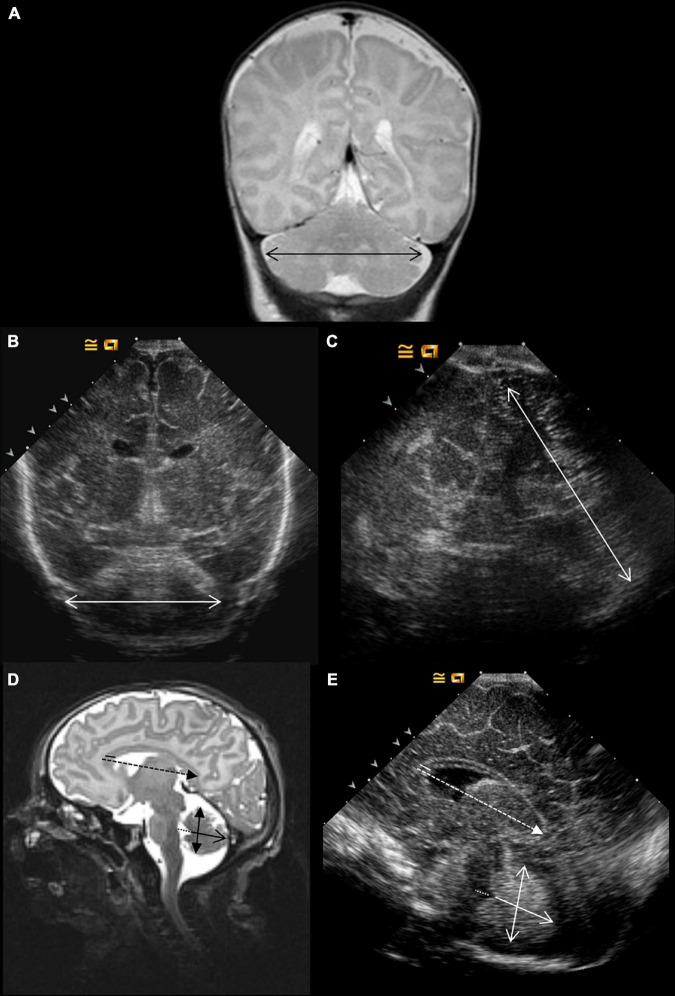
Imaging metric protocol. Coronal and sagittal views. On T2-weighted MRI, the transcerebellar diameter is measured on a coronal view (A). Two acoustic windows were compared on ultrasound: coronal (B) and mastoid planes (C). On T2-weighted MRI (D) and ultrasound, sagittal view (E) were assessed the corpus callosum length (discontinued arrow) and width (plain line), the anteroposterior vermis diameter (plain arrow) and height (double head arrow), and the fourth ventricle diameter (discontinued line).
